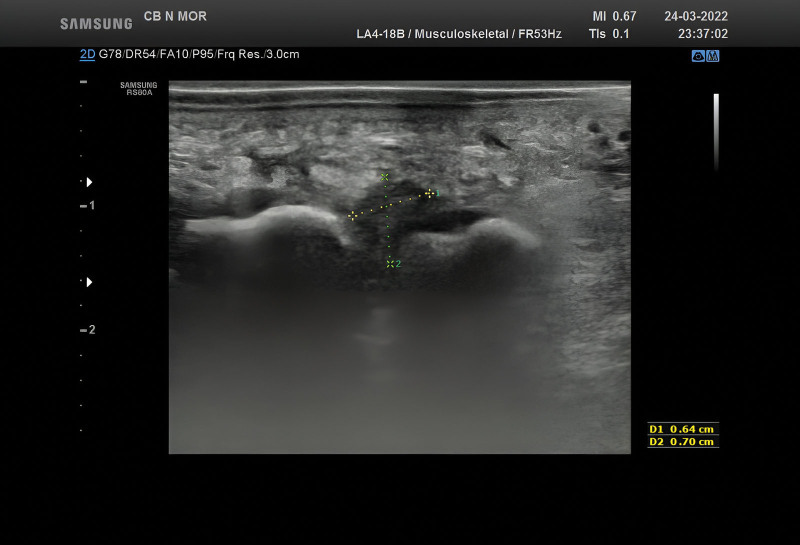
A distinct, well-defined picture measuring 6/7 mm in size, located at the ends of metatarsals III and IV. This image appears as a nodular, hypoechoic, and homogeneous structure, and there is no Doppler signal detected.
